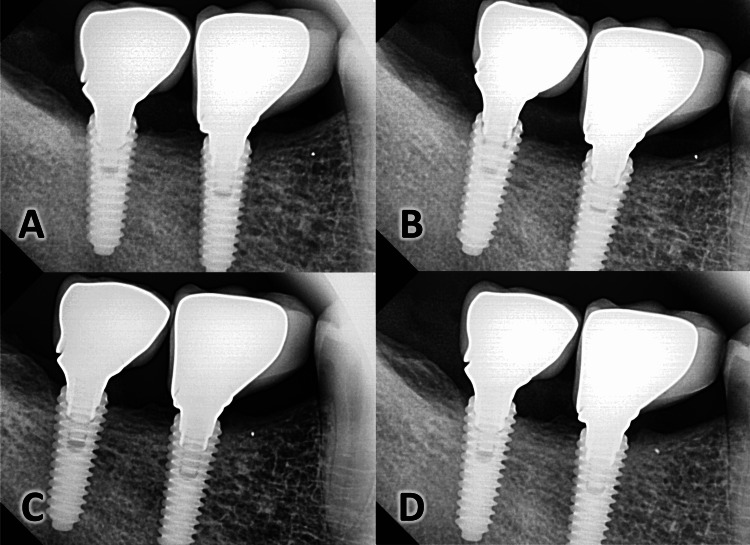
Radiographic assessment of the alveolar bone changes at four assessment times The radiographic appearance of the bone (A) immediately following the fixation of the final prosthesis; (B) after three months; (C) after six months; (D) after one year.
